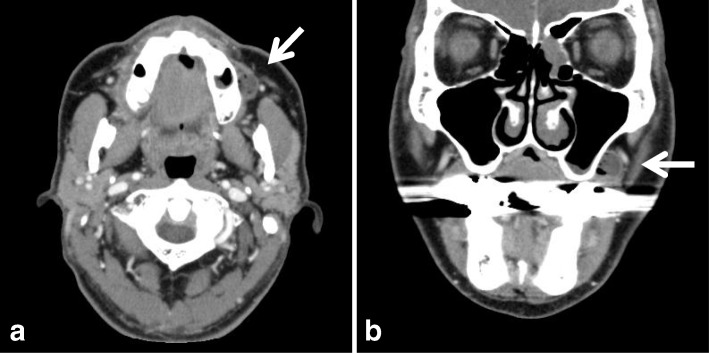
Computed tomography images, a) axial view, b) coronal view, showing a deflection of the buccal mucosa and a cavity containing fluid and air (white arrow)
